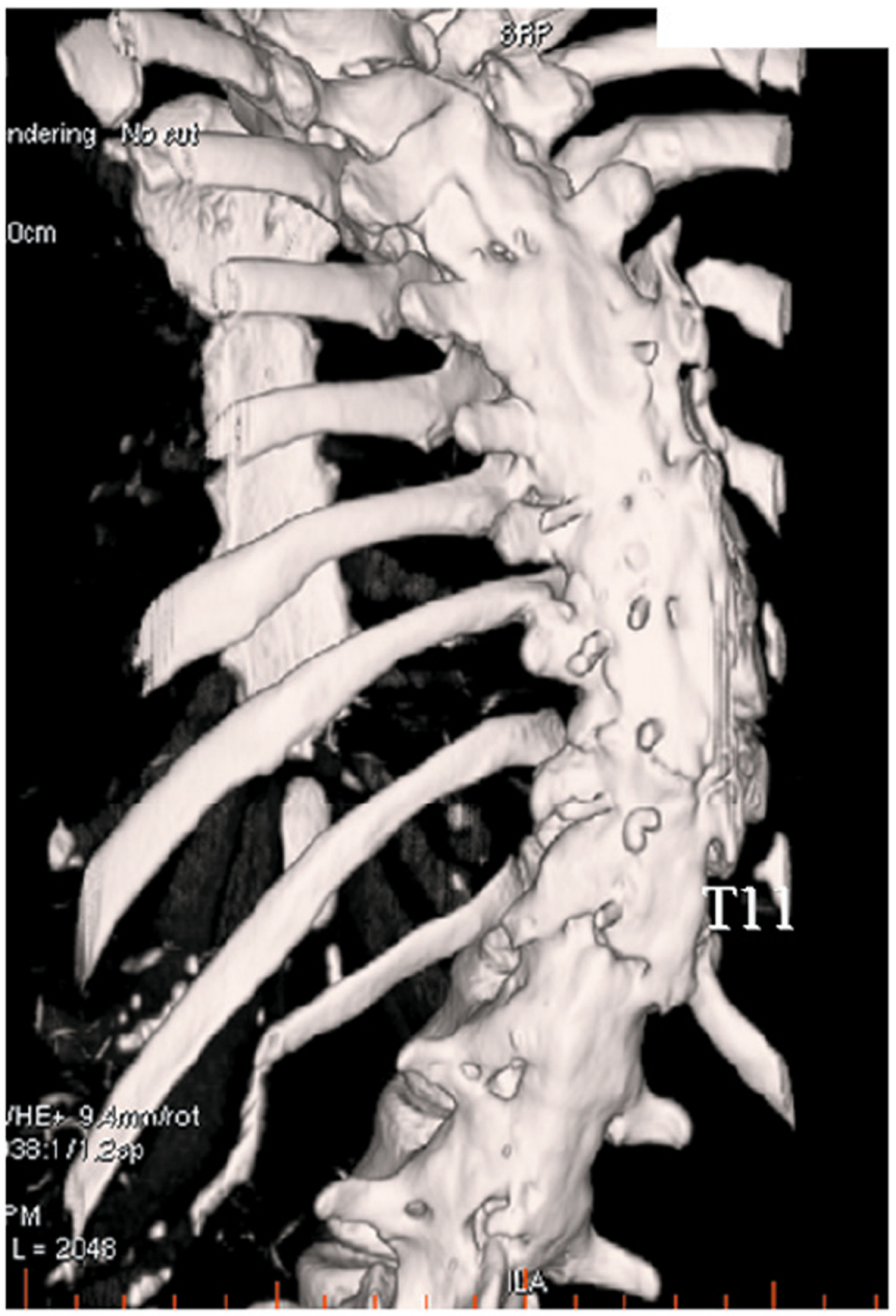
Three-dimensional computed tomography (CT) image prior to reinstrumentation.
